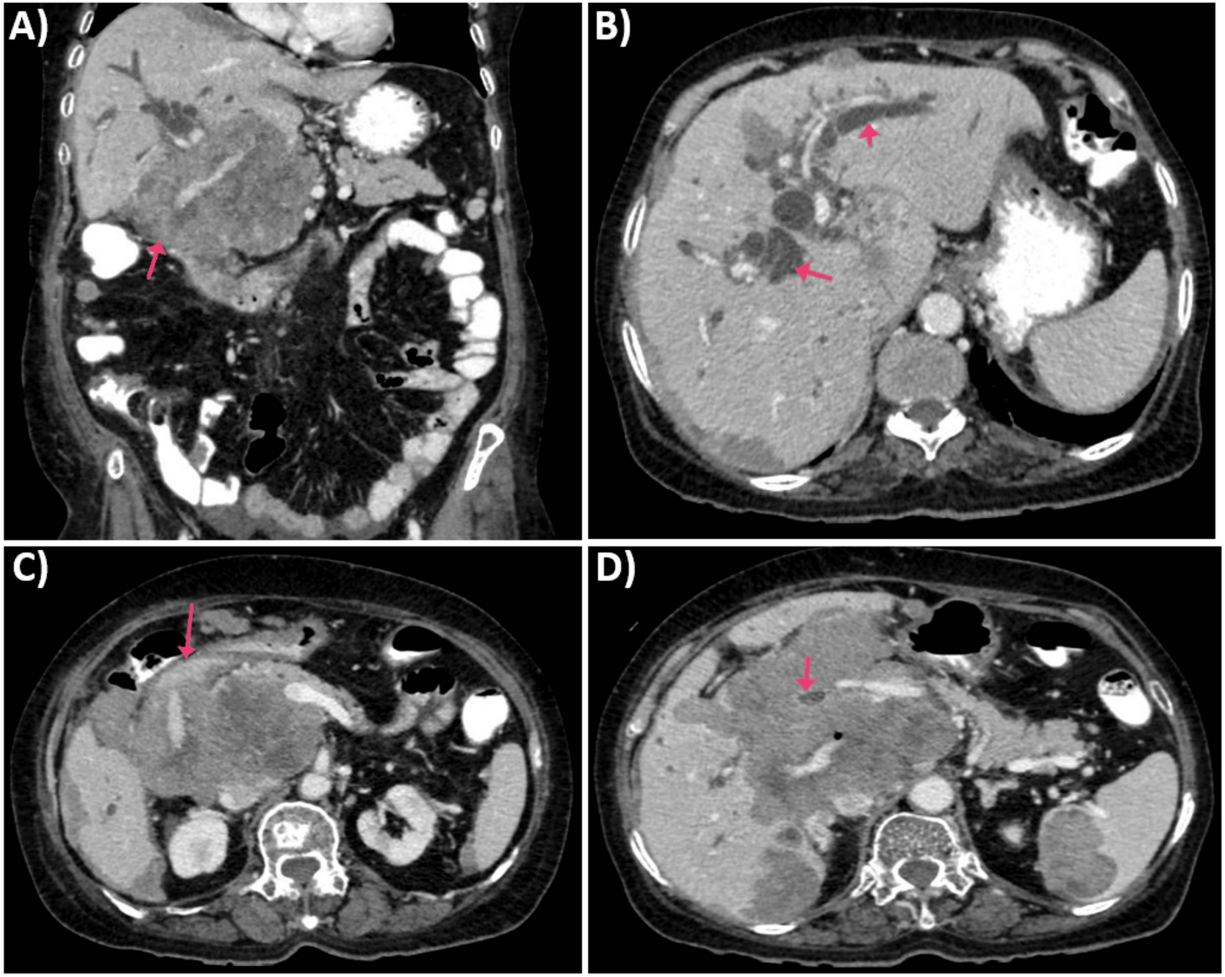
CECT abdomen pelvis: (A) CECT abdomen pelvis, coronal image showing liver metastasis with encroachment of CBD. (B) CECT abdomen pelvis showing intrahepatic biliary radical dilation. (C) CECT abdomen pelvis showing duodenal metastasis. (D) CECT abdomen pelvis showing CBD encasement from liver lesion CECT: contrast-enhanced computed tomography; CBD: common bile duct
